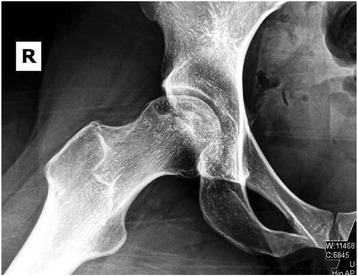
AP view of the right femoral head shows areas of hyperlucency and surrounding sclerosis, as well subtle changes in the shape of the articular surface. The necrosis also spreads into the acetabulum. This x-ray was done just before the patient was referred to a hip revision specialist
